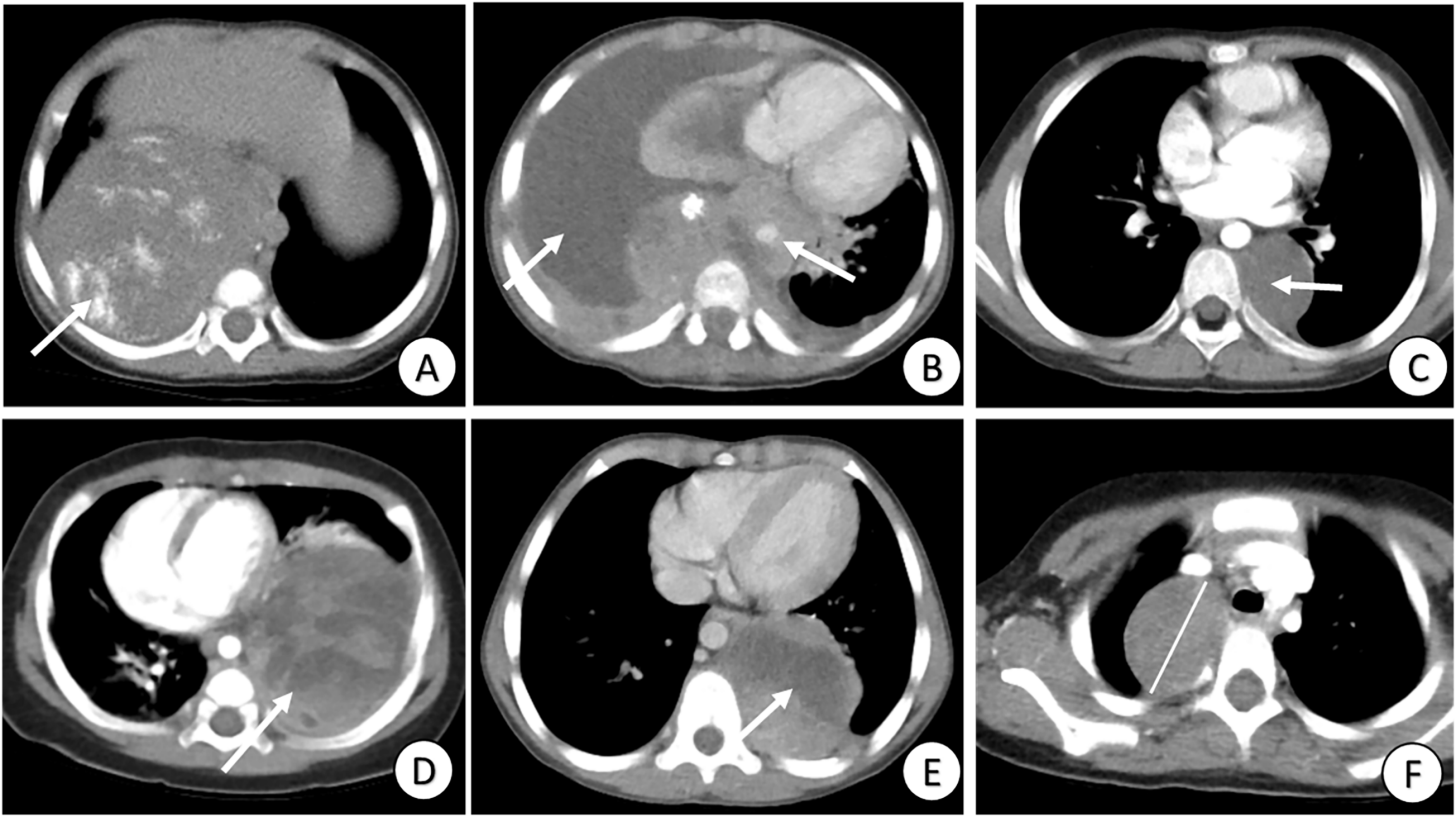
Review of conventional clinical data and CT signs. (A) Tumor with calcification (arrow); (B) Tumor with pleural effusion, infiltration across the midline and vascular wrapping (arrows); (C) Tumor with well-defined margins and heterogeneity (arrow); (D) Tumor with heterogeneity and nonuniform enhancement (arrow); (E) Tumor with necrosis (arrow); and (F) Tumor with maximal diameter = 4.3 cm (line).
